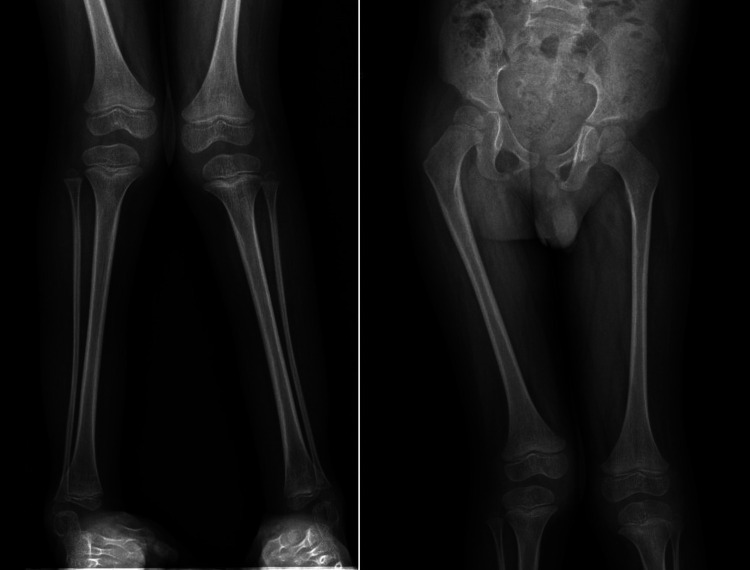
Computed radiography (CR) images of the proband's lower limbs. The patient's lower limbs exhibit pelvic tilt, bilateral knee joint valgus, and bilateral clubfoot.
